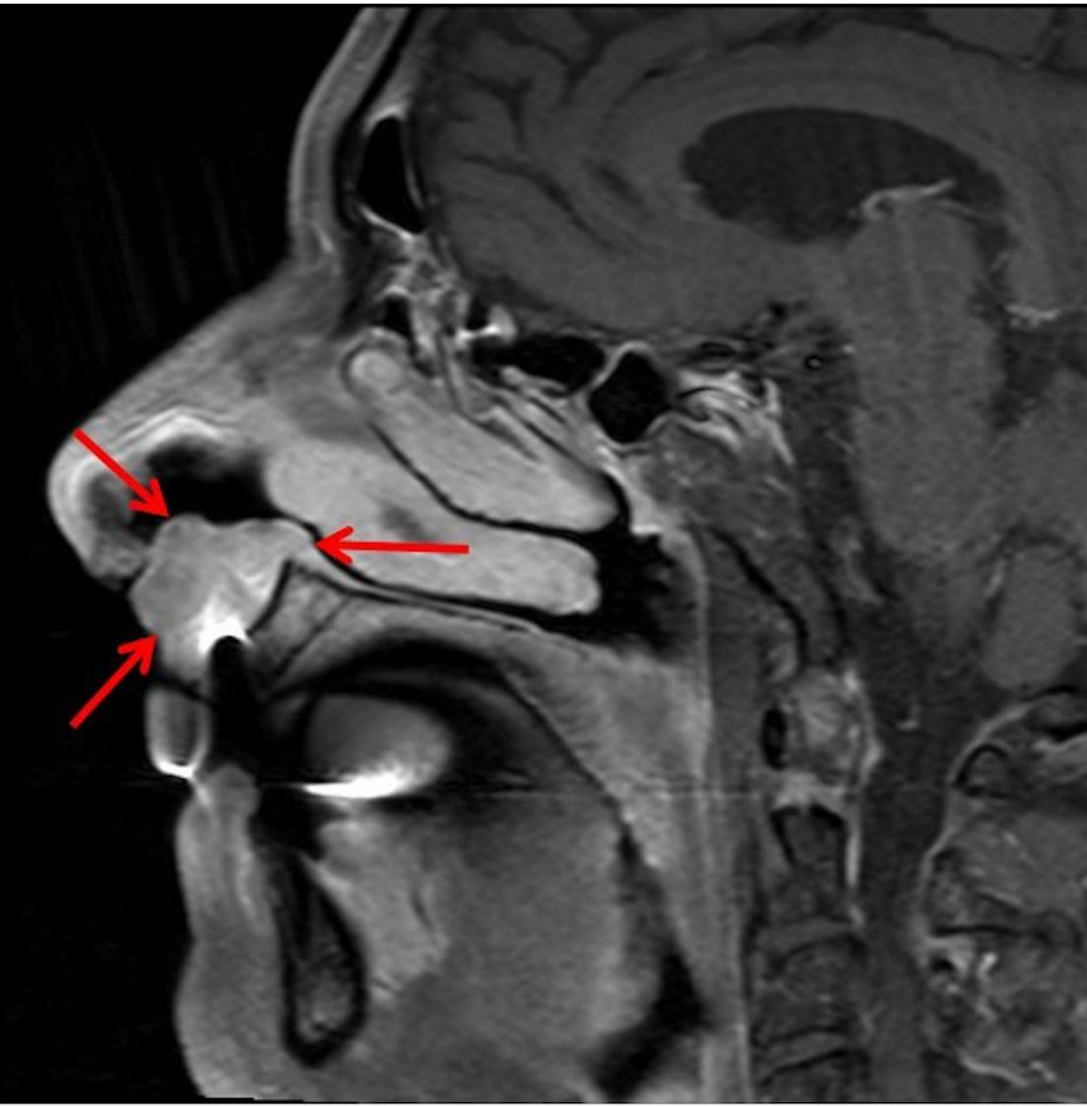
Sagittal slice of the MRI. Representative sagittal slice of MRI showing the extent of the tumor (red arrows). MRI: Magnetic resonance imaging
